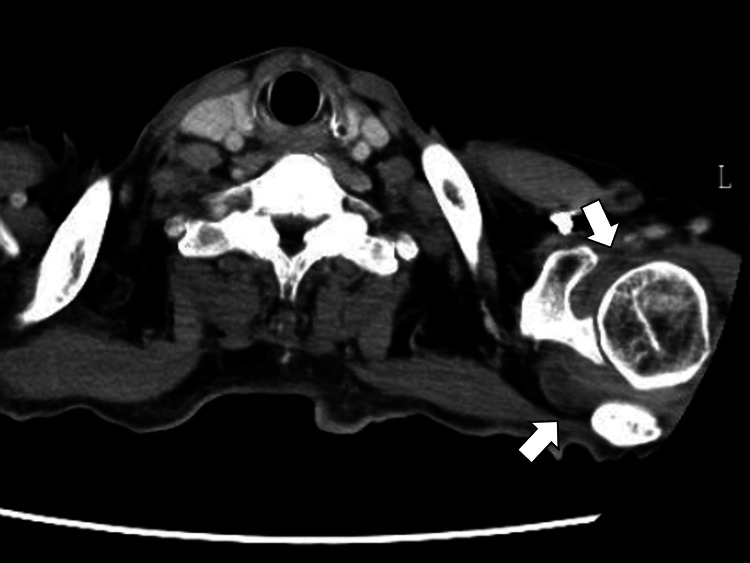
Contrast-enhanced computed tomography showing fluid accumulation in the left acromioclavicular joint (white arrows)
